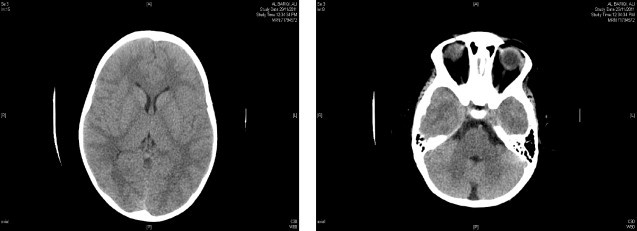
Computed tomography of the brain.
